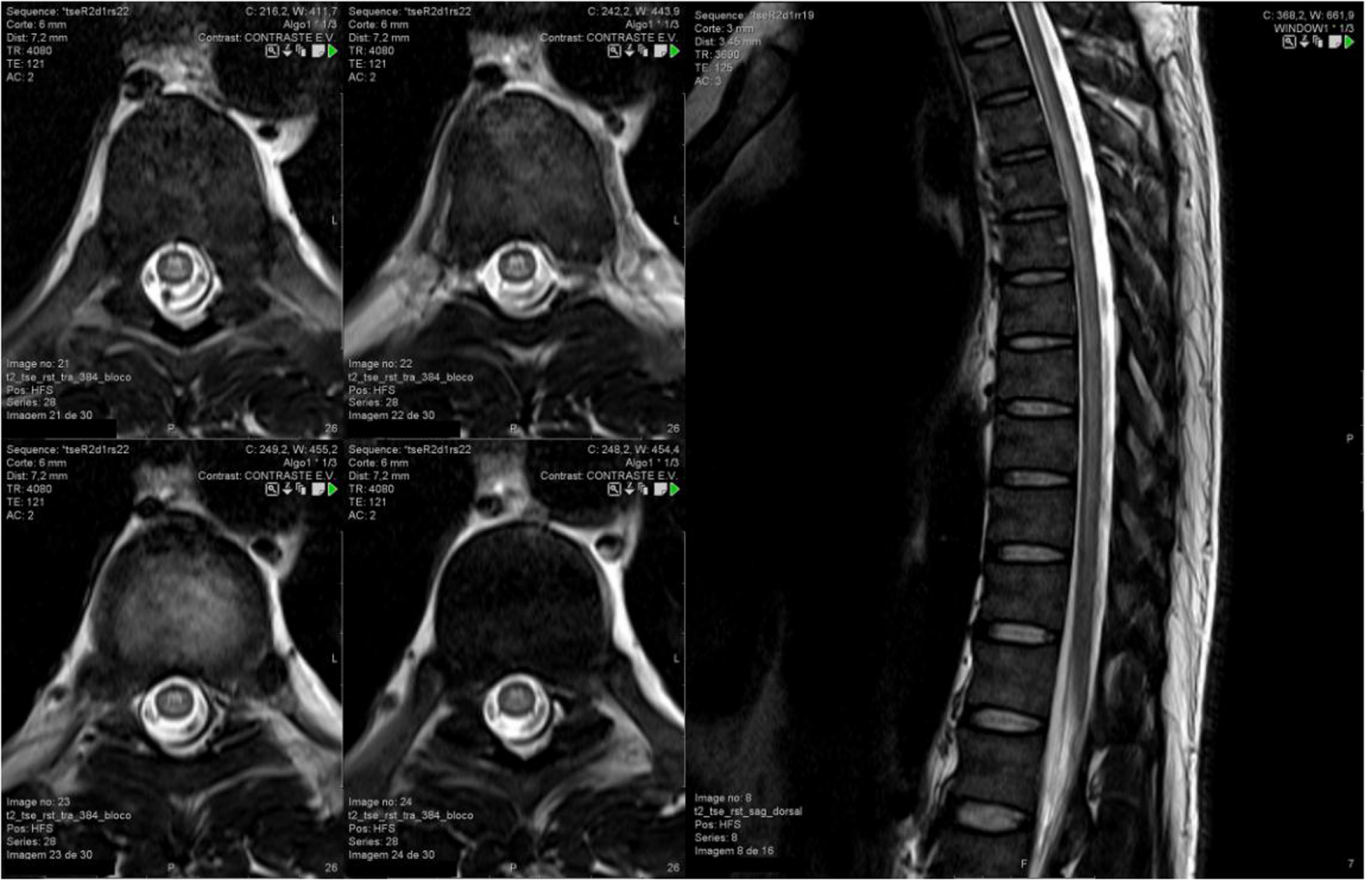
Axial and sagittal T2-weighted magnetic resonance images. Bilateral and symmetrical T2 hyperintensity in the thoracic spinal cord, extending from D2 to D11, predominantly involving the posterior columns. No swelling, atrophy or enhancement were present. Although not specific, these findings might be consistent with HIV myelopathy.
